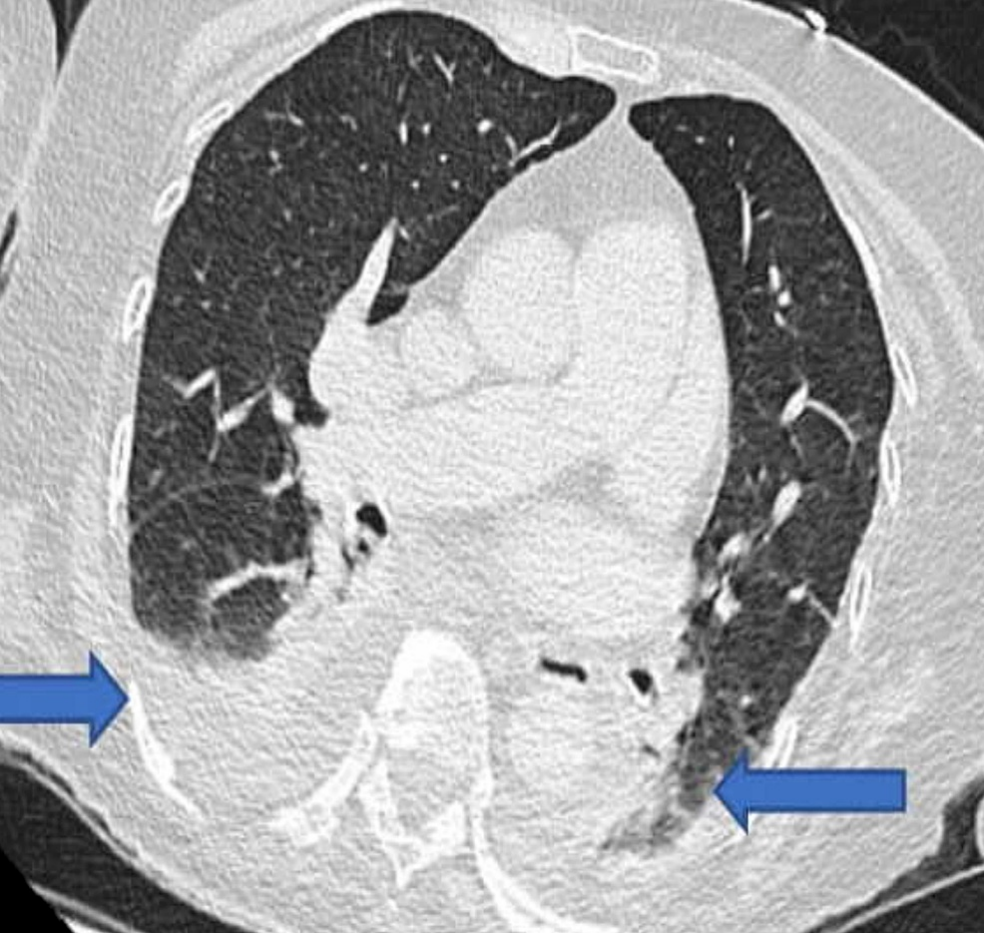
CT of the chest, abdomen and pelvis showing bilateral pleural effusions
